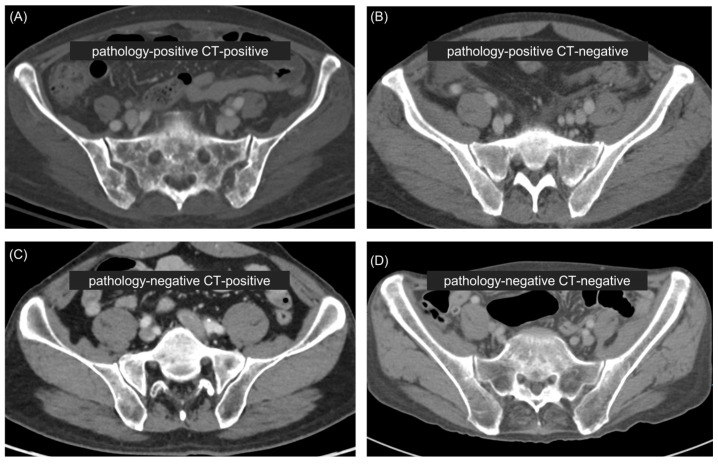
Representative cases. (A) CT image of a 67-year-old female patient shows heterogeneous densities in the sacrum and iliac bone, strongly suggesting bone metastasis. The bone marrow metastasis prediction model predicted bone metastasis based on the radiomic features. Pathological examination of the bone marrow confirmed bone metastasis. (B) CT image of a 55-year-old male patient shows no definite gross marrow changes; however, bone metastasis was pathologically confirmed. The prediction model predicted bone metastasis based on the radiomic features. (C) CT image of a 57-year-old male patient shows suspicious marrow inhomogeneity, but pathological examination confirmed no bone metastasis. The prediction model predicted no metastasis based on the radiomic features. (D) CT image of a 68-year-old male patient shows no marrow changes, and pathological examination confirmed no bone metastasis. The prediction model predicted no metastasis based on the radiomic features.
